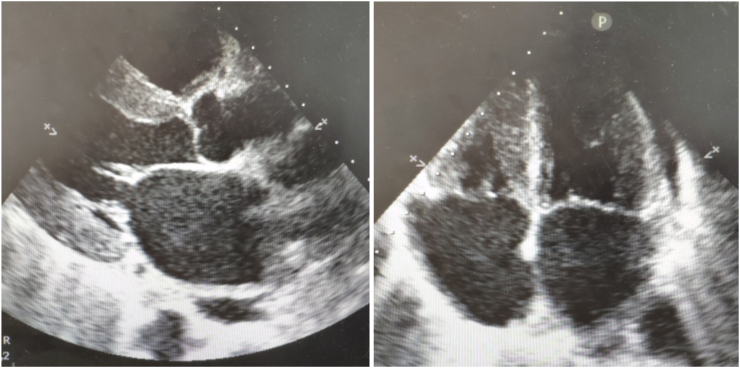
Echocardiography: Parasternal long axis view (A) Four chamber view (B) demonstrating increased thickness with a sparkling granular appearance of the myocardium, bi-atrial dilatation.
